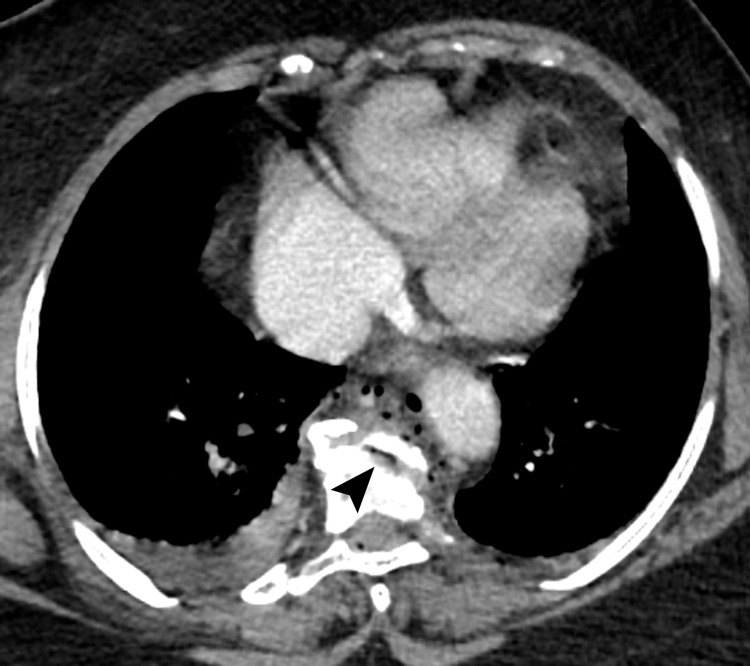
Axial image from CT scan of the chest at the level of the top of the T9 vertebral body shows fracture through the superior endplate containing a small amount of residual air in the disc space (arrowhead). Note multiple small air bubbles in the prevertebral region (pneumomediastinum).
